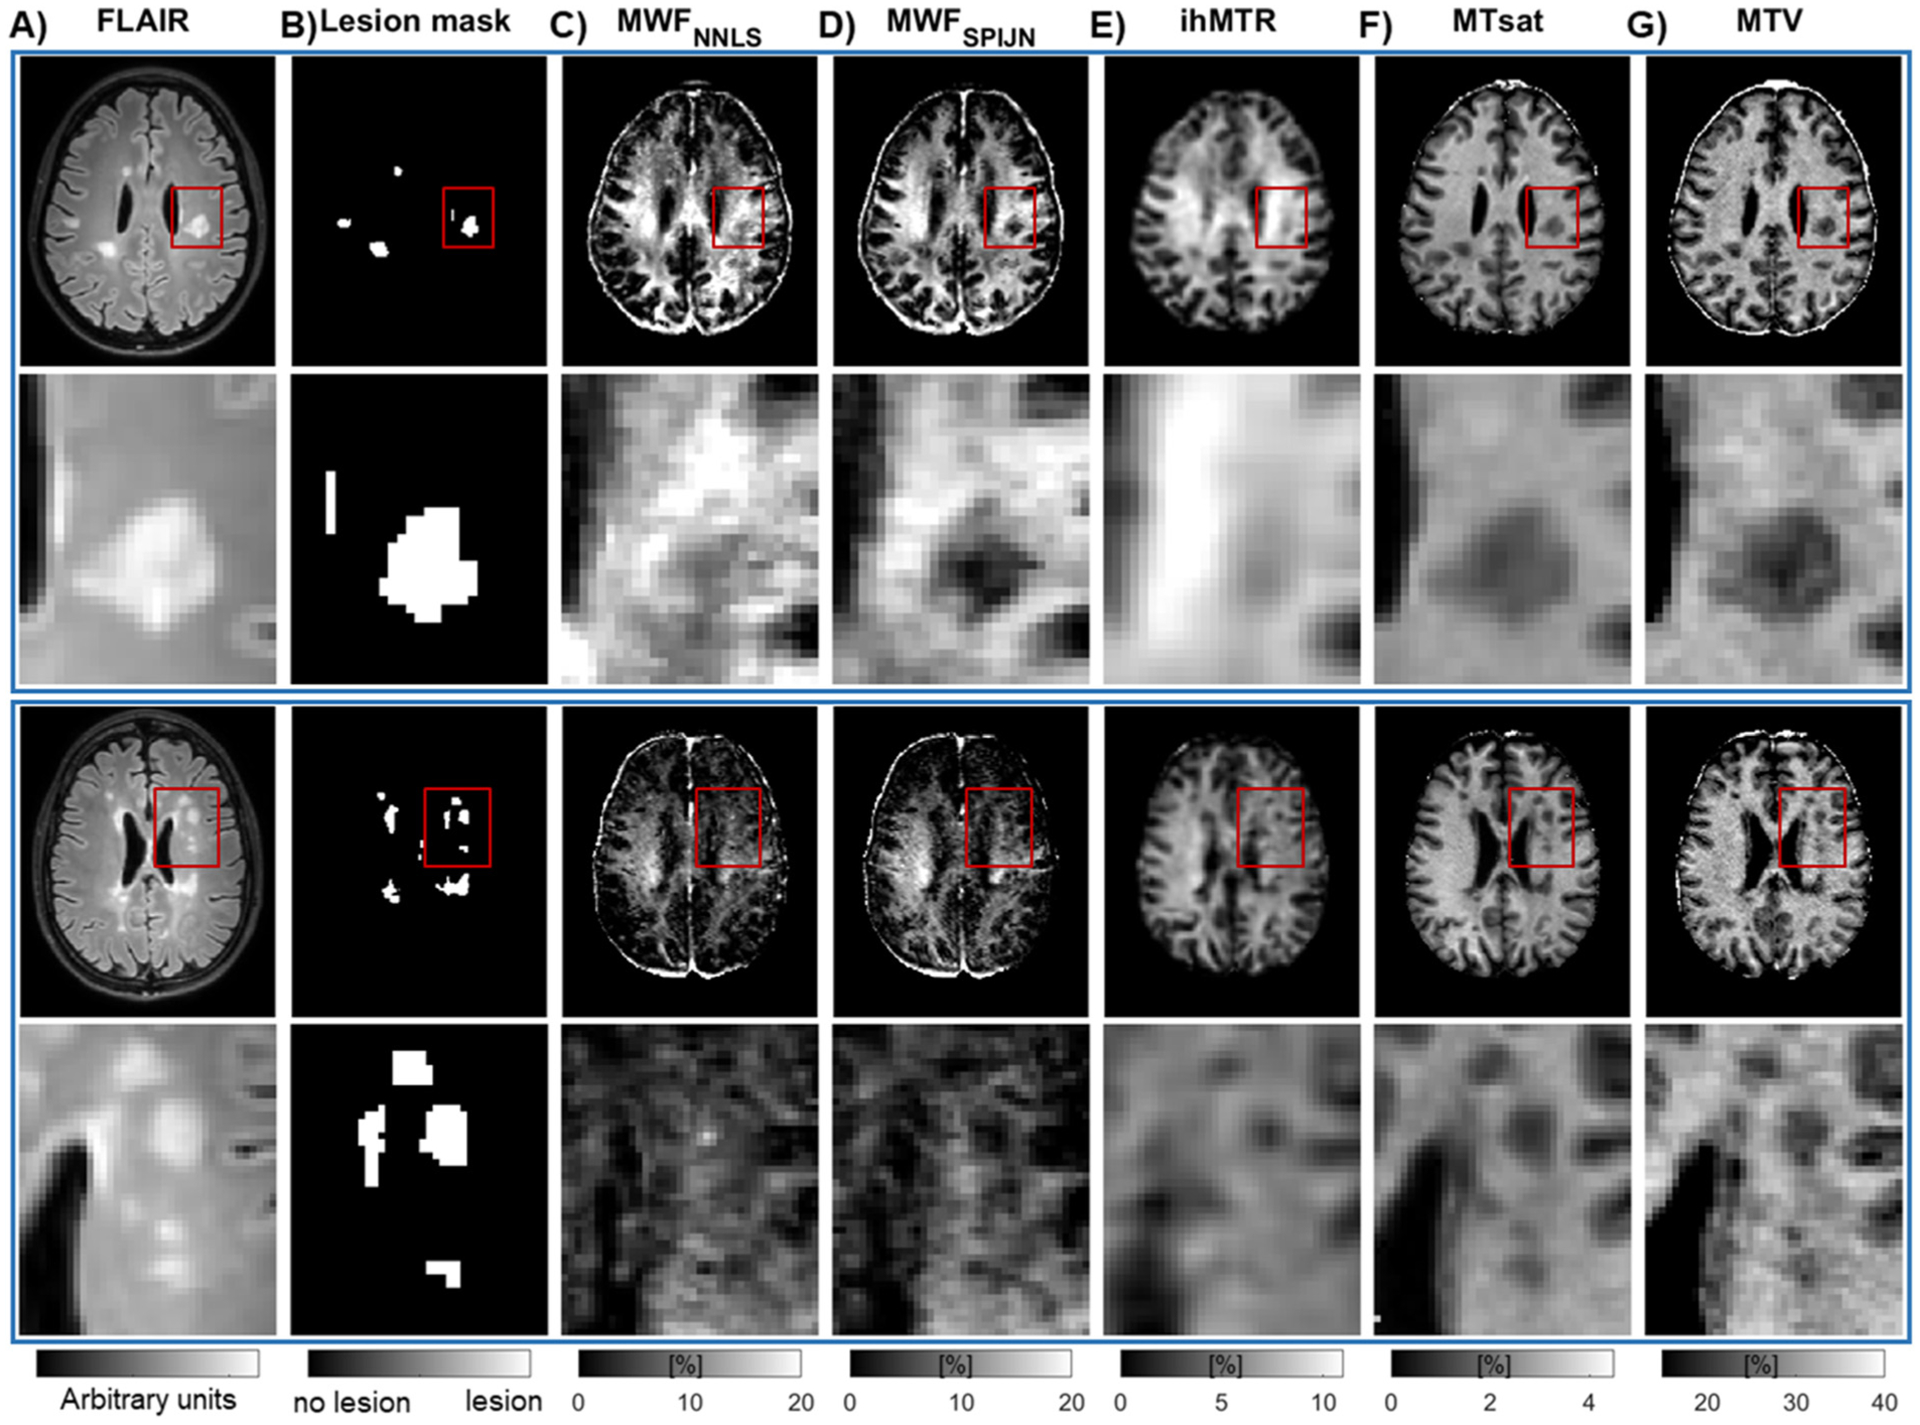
Representative slices of FLAIR (A), lesion segmentations (B), and maps of myelin-sensitive markers (C-G) from two MS patients, including expanded regions. Myelin-sensitive markers include two reconstructions of the myelin water fraction, MWF_NNLS_ (C) and MWF_SPIJN_ (D), inhomogeneous magnetization transfer ratio (ihMTR) (E), MT saturation (MTsat) (F), and macromolecular tissue volume (MTV) (G). Red rectangles in the first and third rows indicate the location of the zoomed-in regions in the second and last rows.
